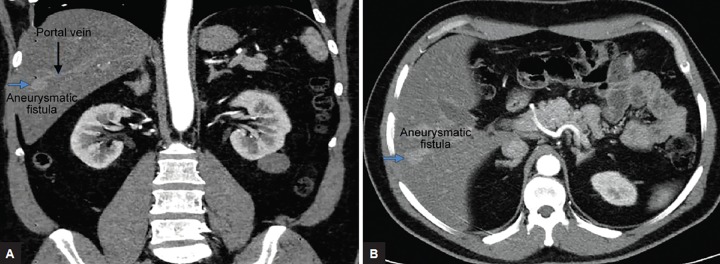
Coronal (A) and axial (B) CTA images reveal an aneurysmatic fistula (blue arrow) approximately 2 cm in size in the intersection of segment 5 to 6 of the right lobe, between the right portal vein (black arrow) and the right hepatic vein branches in the liver
